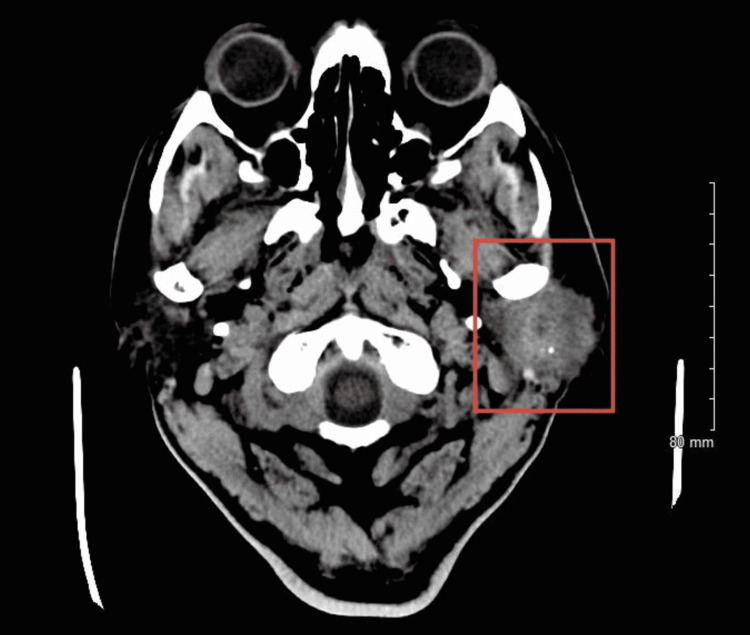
CT soft tissue neck with contrast showing salivary gland tumor centered within the left parotid gland with stranding of the surrounding subcutaneous tissues including the preauricular area as well as inflammatory changes extending toward the cartilaginous segment of the left external auditory canal with associated narrowing.
